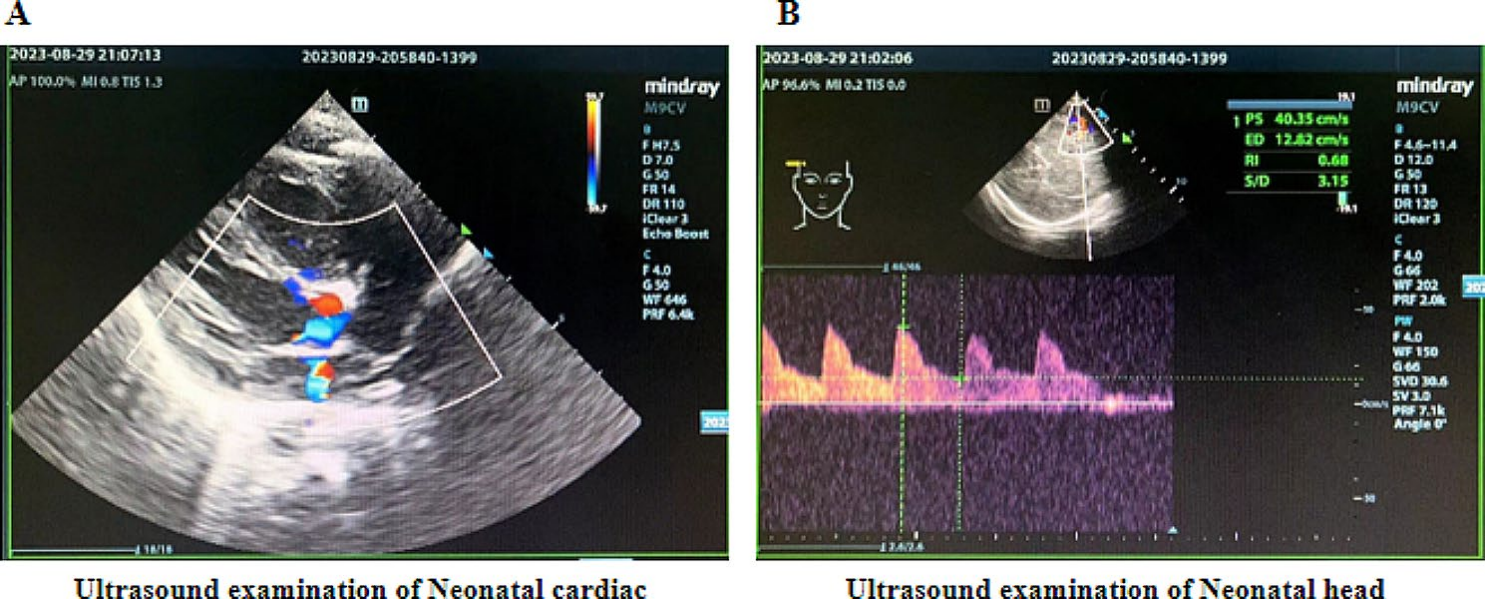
Neonatal ultrasound examination. (A) The ultrasound examination of the newborn’s heart showed no abnormalities. (B) The ultrasound examination of the newborn’s head shows no abnormalities
